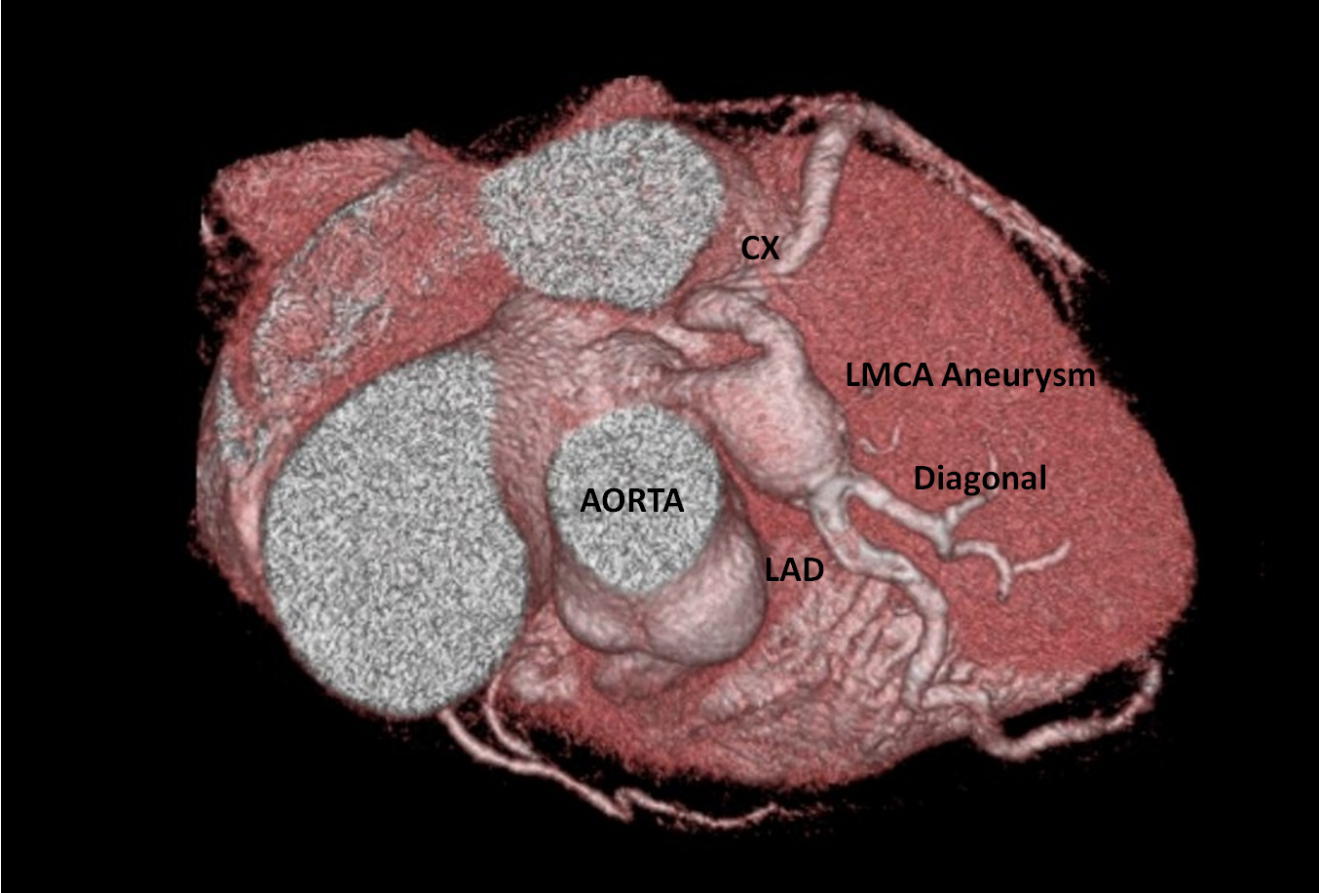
Three-dimensional volume-rendering computed tomography, showing a giant aneurysm of the left main coronary artery.
